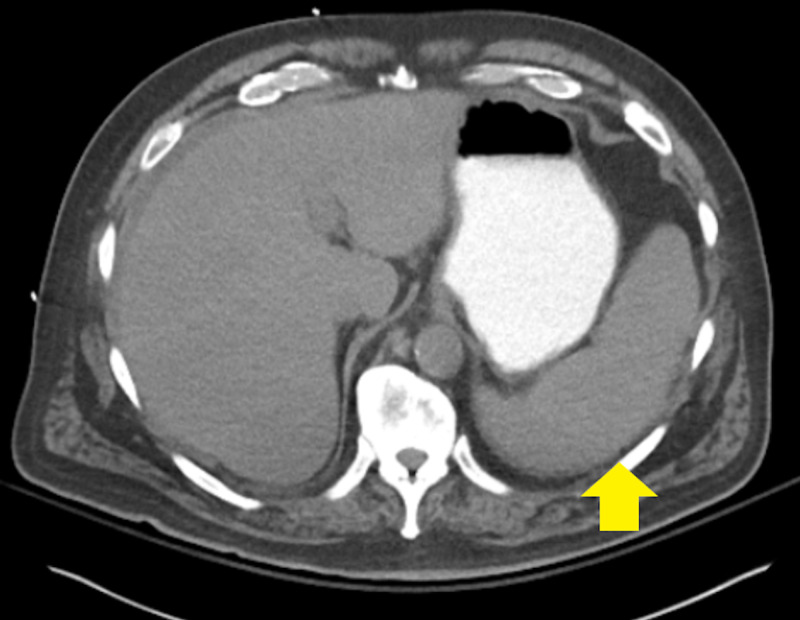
Computed tomography of the abdomen revealed mild splenomegaly in the patient, a common finding in chronic lymphocytic leukemia
